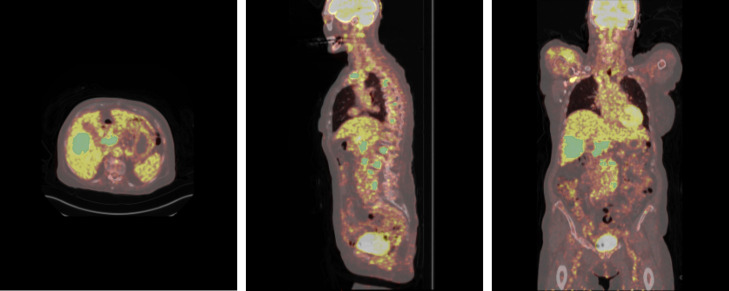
PET/CT scan from the AutoPET dataset, with manual annotations of lymphoma lesions highlighted in green. From left to right: axial, coronal, and sagittal views.
